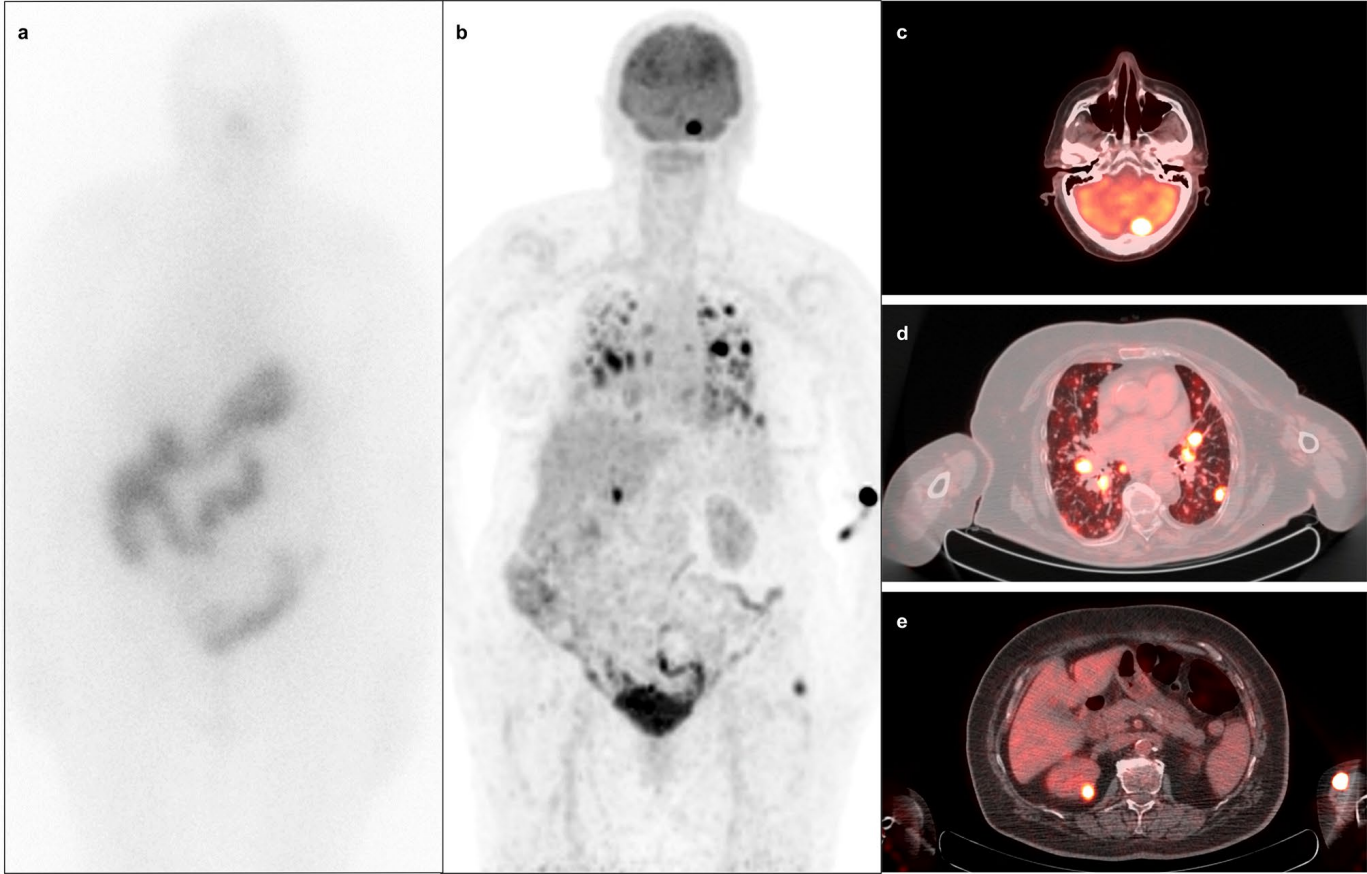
A 79-year-old female presented with high-risk papillary thyroid carcinoma after total thyroidectomy and central neck lymph node dissection, radiotherapy to the neck and upper mediastinum, and multiple cycles of [^131^I]I therapy with a total activity of 22.6 GBq. Post-therapeutic [^131^I]I WBS performed during the last radioiodine treatment was negative (a). The patient had an [^18^F]FDG PET/CT scan performed due to elevated thyroglobulin levels (202.3 ng/ml), which revealed [^18^F]FDG-avid metastatic lesions in the cerebellum, lungs, and right kidney (b-e)
